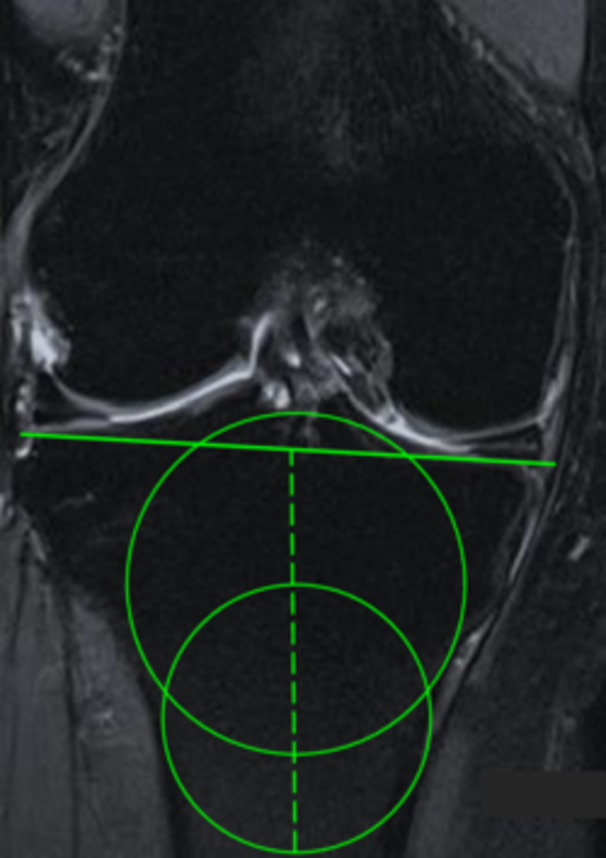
Magnetic resonance image of the medial proximal tibial angle. On the coronal plane in which the bilateral tibial eminences are clearly visualized, two circles are drawn, each tangent to the cortical margins of the bone. The line connecting the centres of these circles is defined as the tibial axis (long dashed line). The medial proximal tibial angle is measured as the medial angle between the tibial axis and the solid line connecting the most superior medial and lateral cortical edges.
